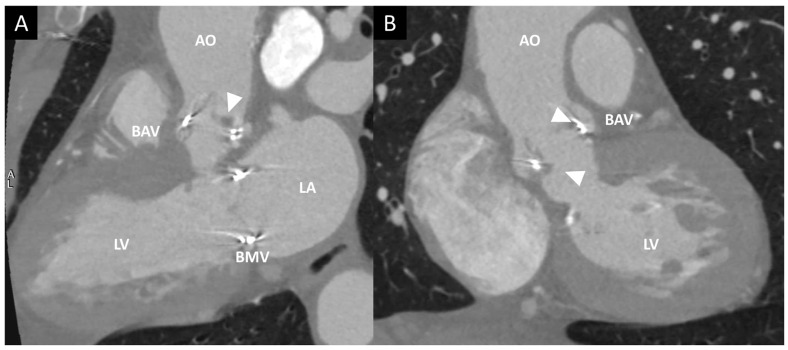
Contrast CT in a patient with endocarditis of a bioprosthetic aortic valve (BAV). (A,B) Multiplanar reconstruction of LV long axis (A) and LV short axis (B). Arrowheads show the presence of hypodense masses adherent to BAV leaflets. AO, aorta; BMV, bioprosthetic mitral valve; LA, left atrium; LV, left ventricle.
